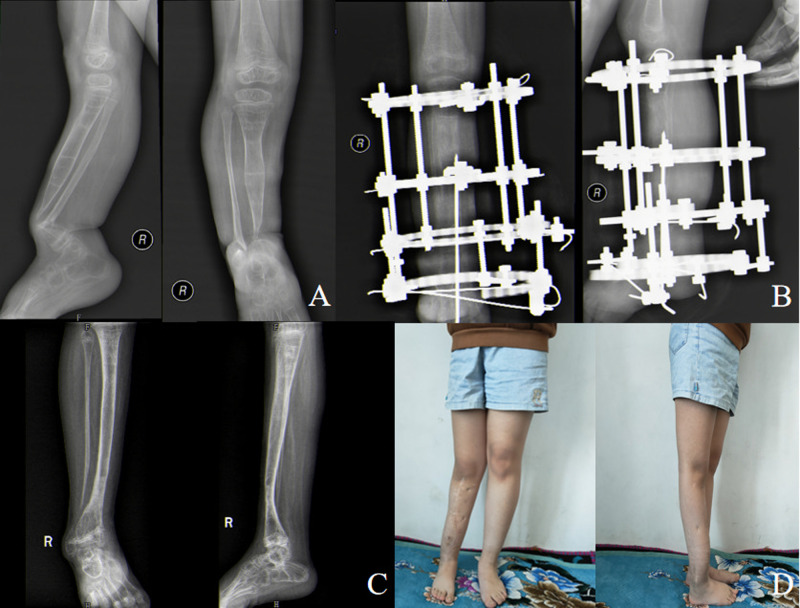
Typical case of a 5.5-year-old girl with congenital pseudarthrosis of the tibia. (A) Preoperative anteroposterior and lateral X-ray showing Crawford type IV CPT and intact fibula. (B) Anteroposterior and lateral X-ray films at 98 days after operation, showing good consolidation of distracted region. (C) Anteroposterior and lateral radiographs at 40 months after removal of the external fixator, showing a well-aligned tibia and bone union. Thirty-six months after the external fixator was removed, the intramedullary K-wire was removed in order to restore the range of motion of the ankle joint. (D) Forty-one months after removal of the external fixator, the patient’s standing and walking function recovered.
